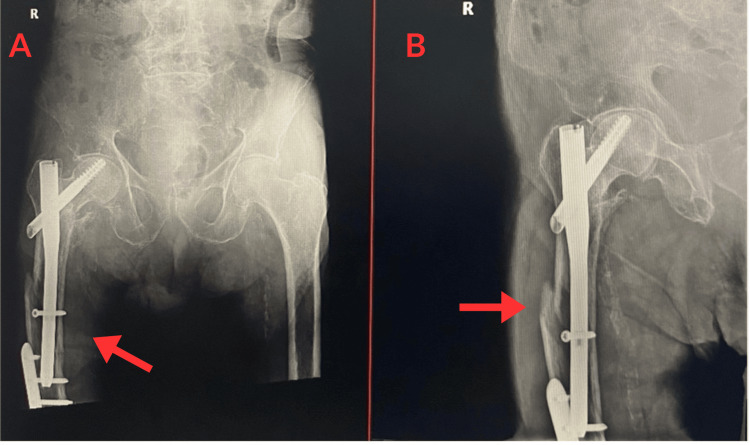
X-ray of the right hip (AP view), 17 July 2025, revealing an acute fracture in the proximal third of the right femur adjacent to the existing TFNA hardware. (A) and (B) demonstrate an acute fracture in the proximal third of the right femur (red arrows) adjacent to the TFNA hardware. Both images show the same fracture from slightly different exposures to enhance visualization of the fracture line.
